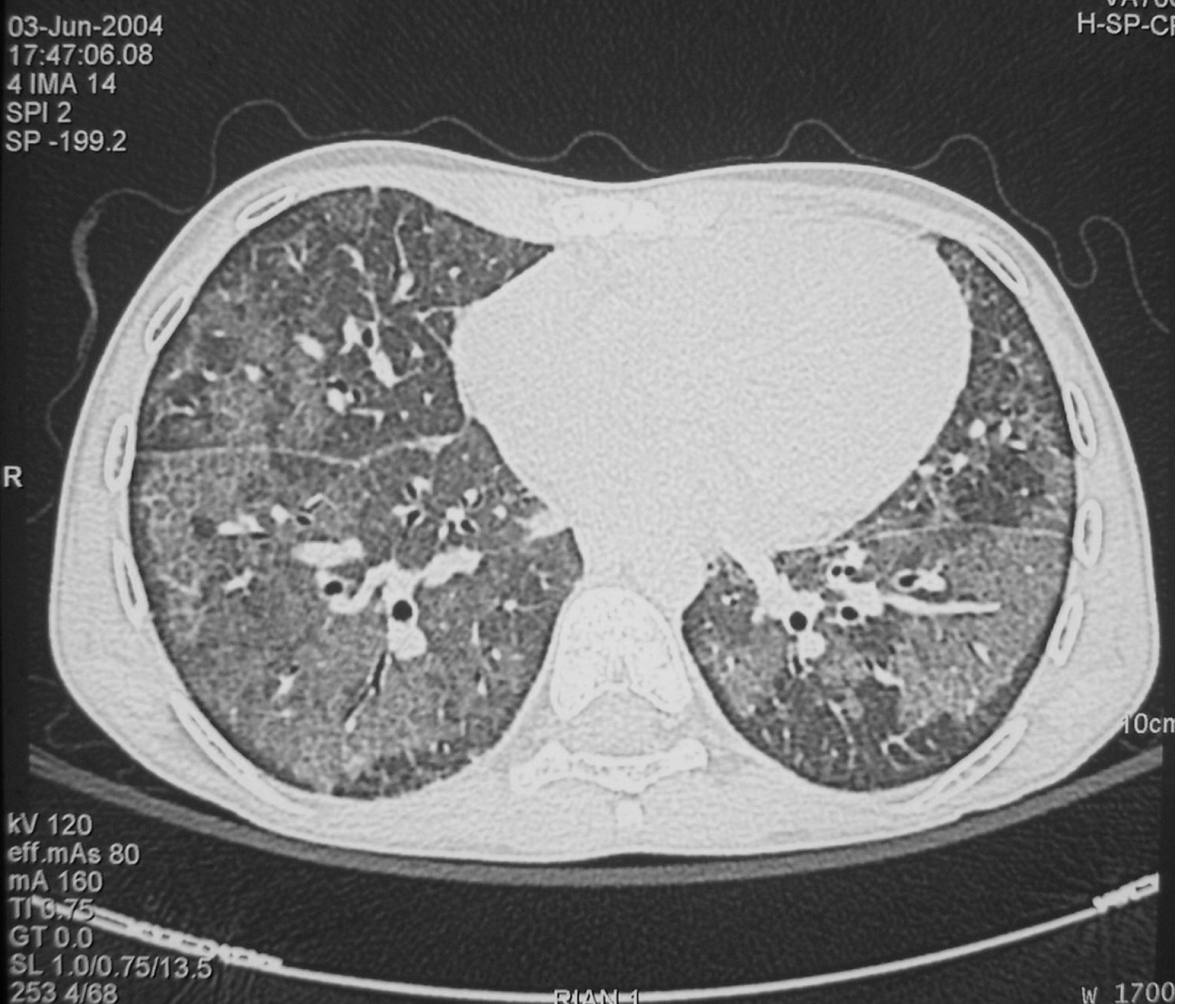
Chest HRCT performed 1 day before WLL. Areas of ground glass attenuation with concomitant interlobular septa thickening are evident, alternated with areas of normal lung (crazy paving pattern).
